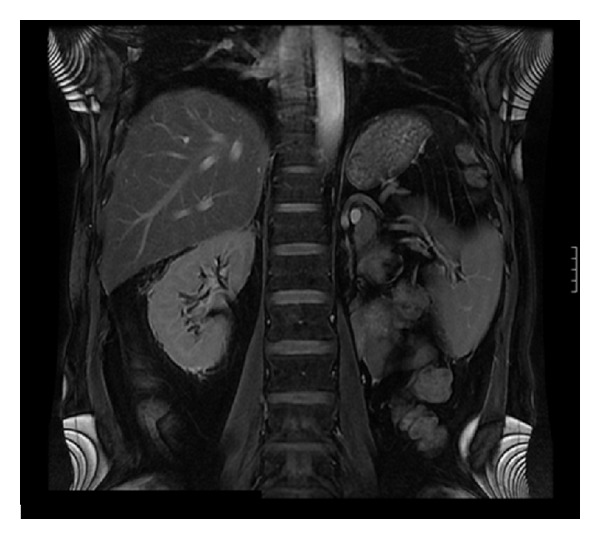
Postoperative magnetic resonance imaging (MRI) of the patient (6 months of follow up).
